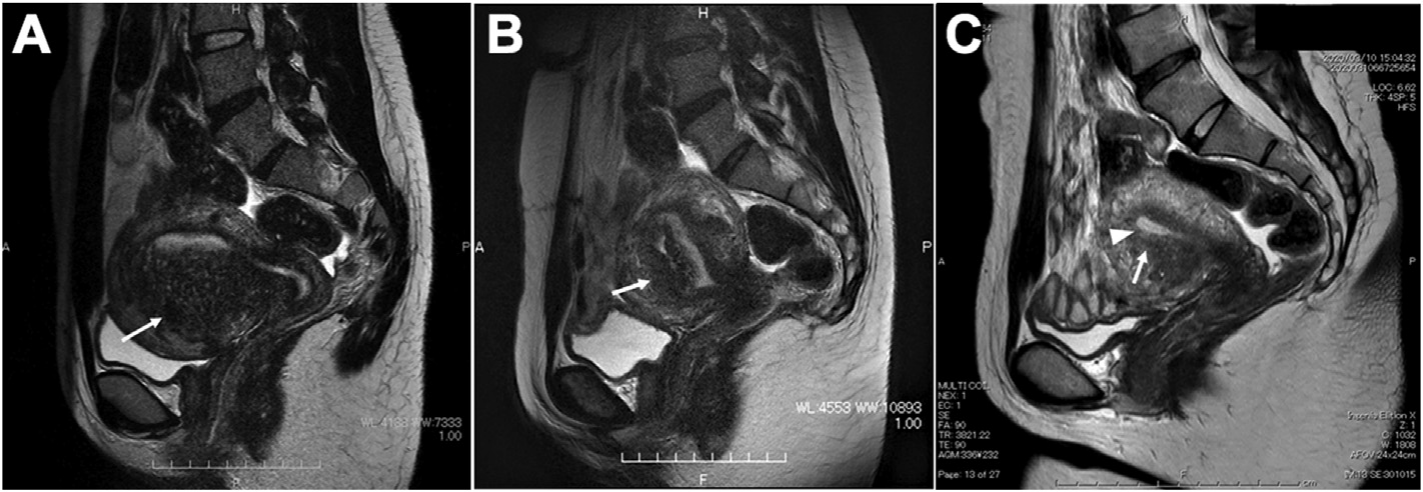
Magnetic resonance imaging (MRI) of pre- and post-laparoscopic adenomyomectomy. A: Sagittal T2-weighted preoperative pelvic MRI. Image shows substantial adenomyosis in the anterior myometrium with a remarkably thickened junctional zone (white arrows). B: Sagittal T2-weighted postoperative pelvic MRI (4 weeks later). Image shows no residual adenomyosis. Note the complete uterine wound-healing (white arrow). C: Sagittal T2-weighted postoperative pelvic MRI (12 weeks later). Image shows normal thickness of the junctional zone (white arrow) and a regular endometrial cavity with no defect visible (arrowhead).
